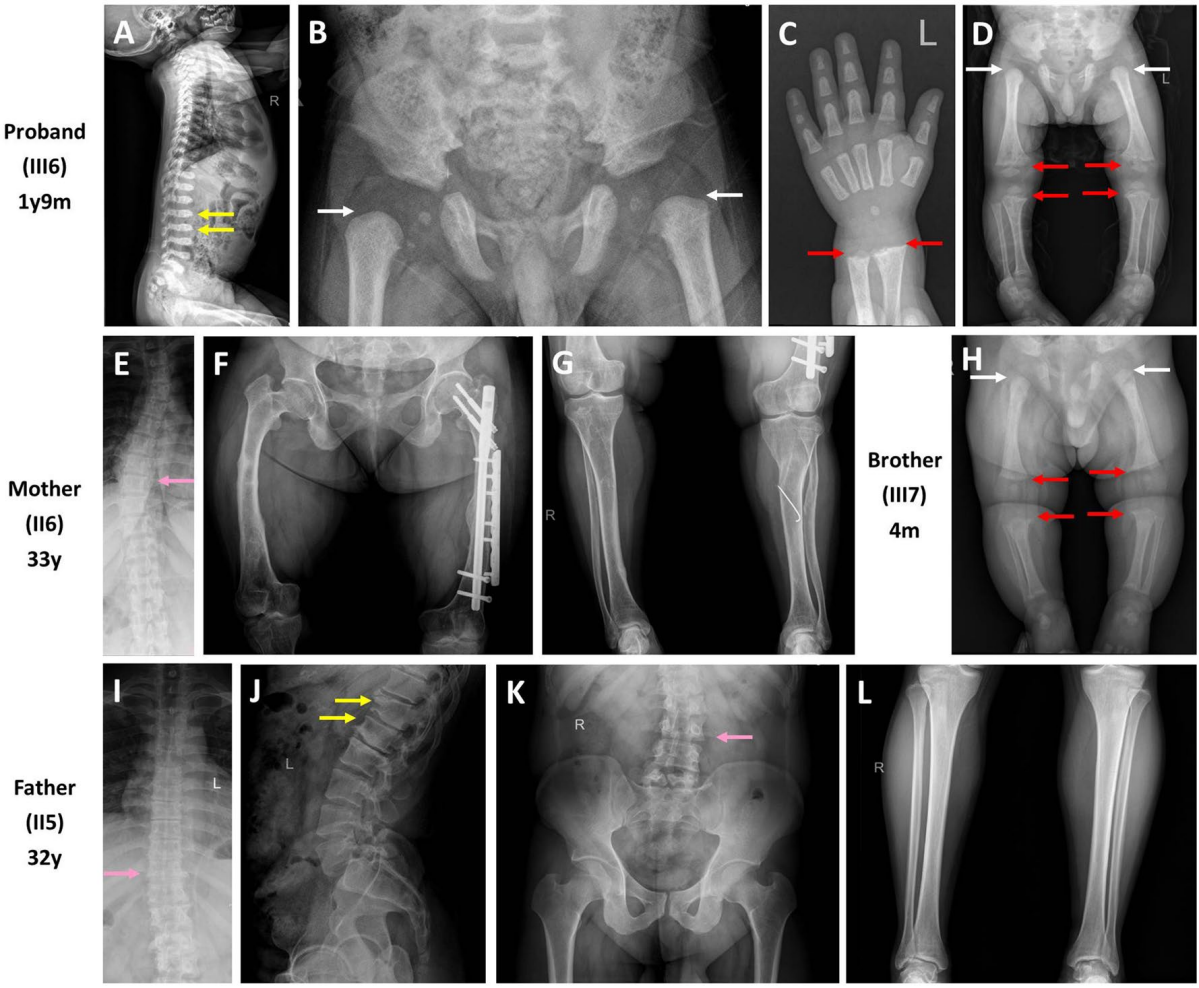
The skeletal X-ray images of the four core patients in the enrolled family. A–D. The skeletal X-ray images of the proband (III6). E–G. The skeletal X-ray images of the mother (II6). H. The skeletal X-ray images of the brother (III7). I–L. The skeletal X-ray images of the father (II5). The yellow arrow indicates flattened and transverse bottles-like vertebral body, the white arrow indicates small epiphyses of the femoral heads, the red arrow indicates widened and brush-like metaphyses, and the pink arrow indicates scoliosis
